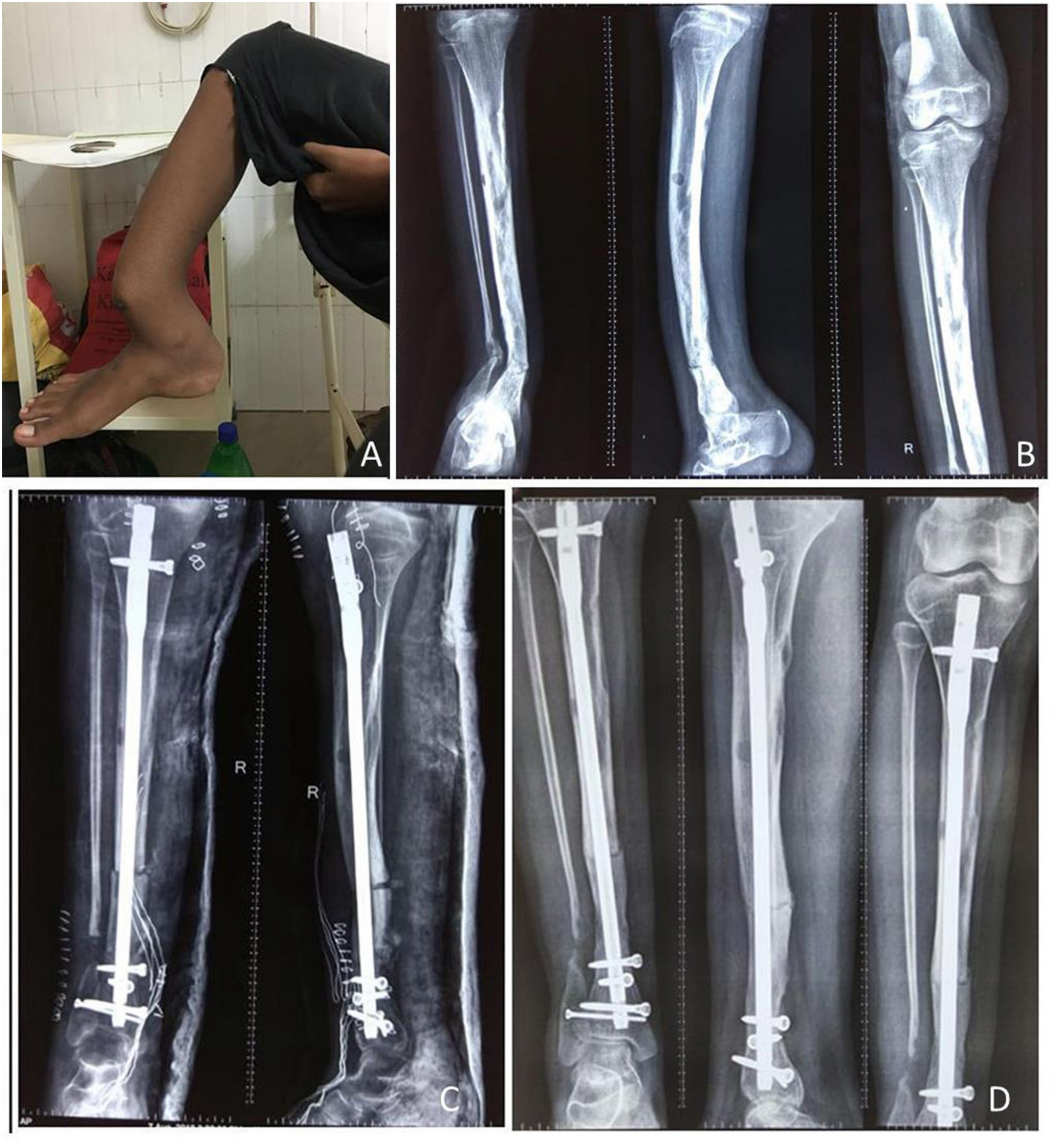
A 17-year-old adolescent girl presented with PFD involving the right tibial shaft and fibula (A,B). After 2 months of zoledronic acid infusion, corrective osteotomy and intramedullary nailing was performed (C), follow-up radiograph at 6 months shows complete healing of the lesion (D).
